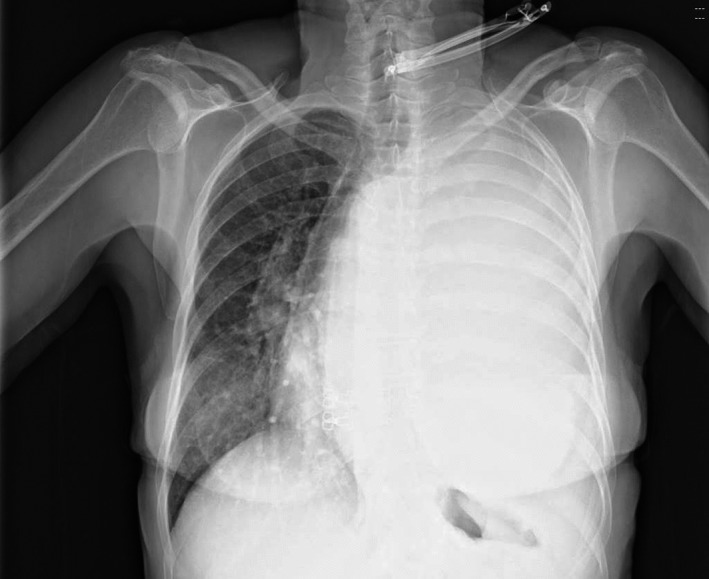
Chest radiography shows a large pleural effusion in the left thorax and mediastinal shifting
